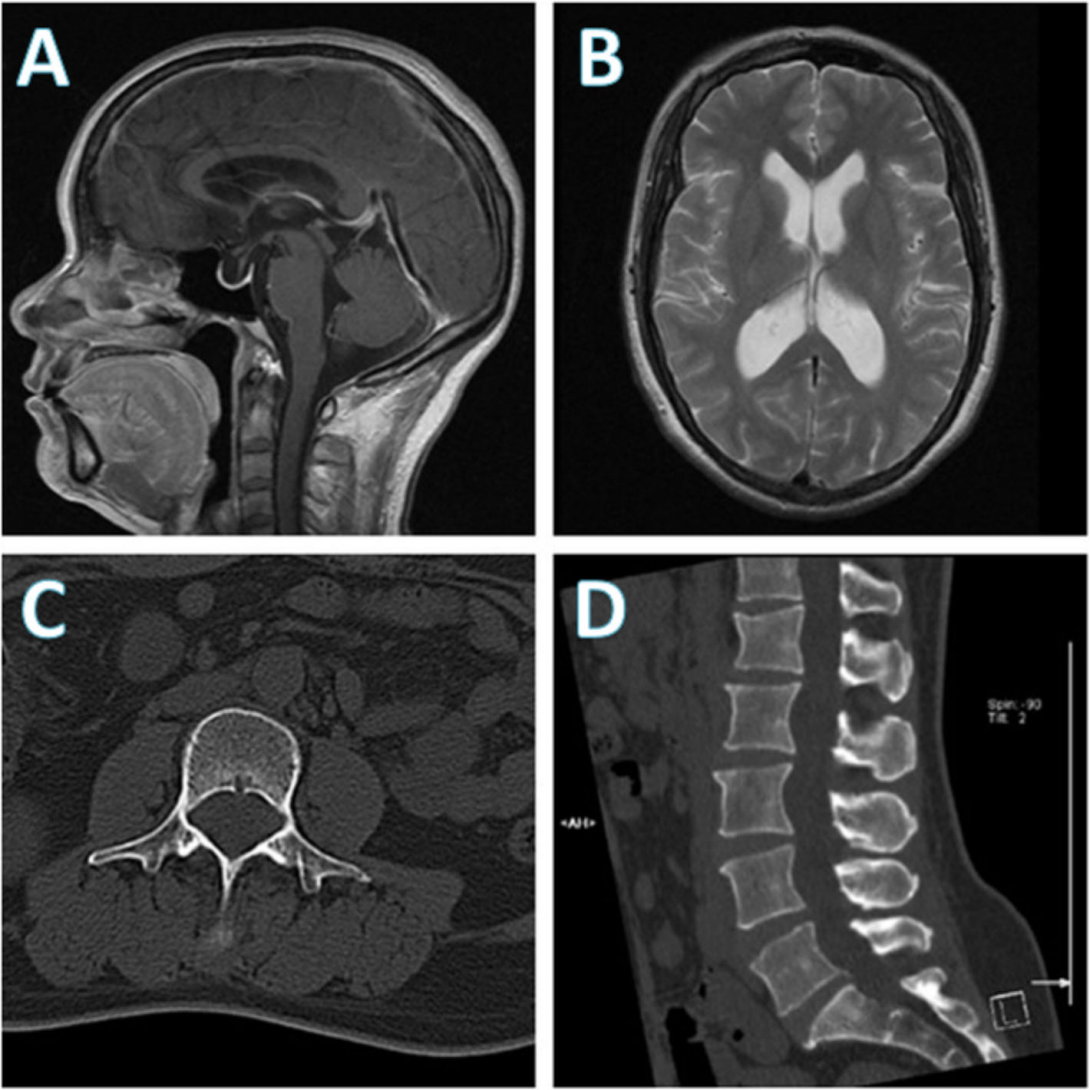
Brain magnetic resonance imaging (MRI) and lumbar computed tomography (CT) performed during the patient’s first admission. a Brain MRI demonstrated mild enlargement of the supratentorial ventricle, b the abnormal sign of vacuolar sella in the right optic-radiation of lateral thalamus, bilateral medial temporal lobes, and the insular lobes. c, d No abnormalities were found in lumbar CT at first; however, a retrospective review of the spinal CT scan (d) showed the evidence of enlarged neural foramina and mild vertebral scalloping which suggested a long-standing intradural tumor such as schwannoma
